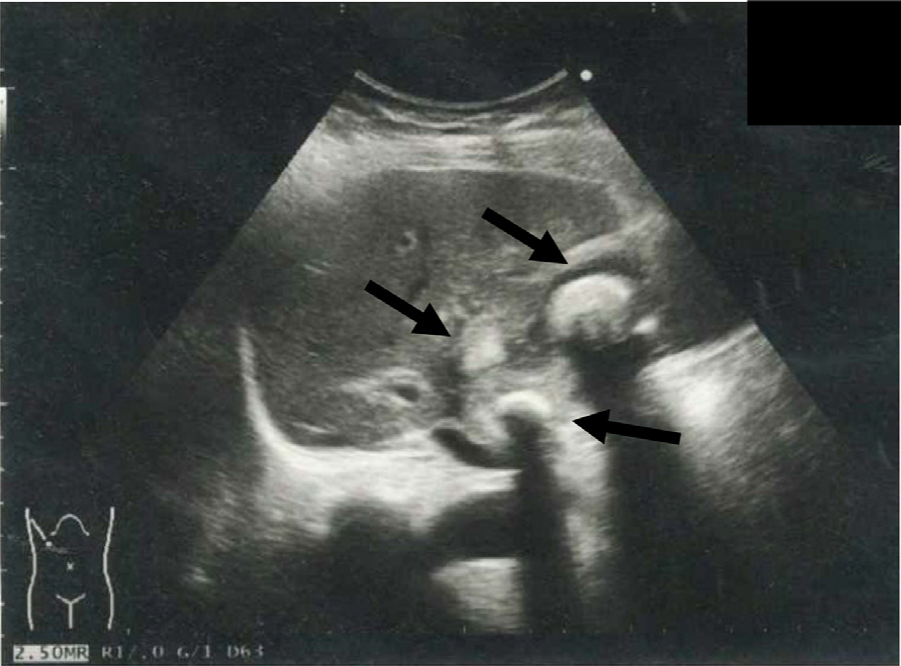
Abdominal ultrasonography before PTGBD shows high echogenic multiple stones (arrow) in the gallbladder. PTGBD, percutaneous transhepatic gallbladder drainage.
